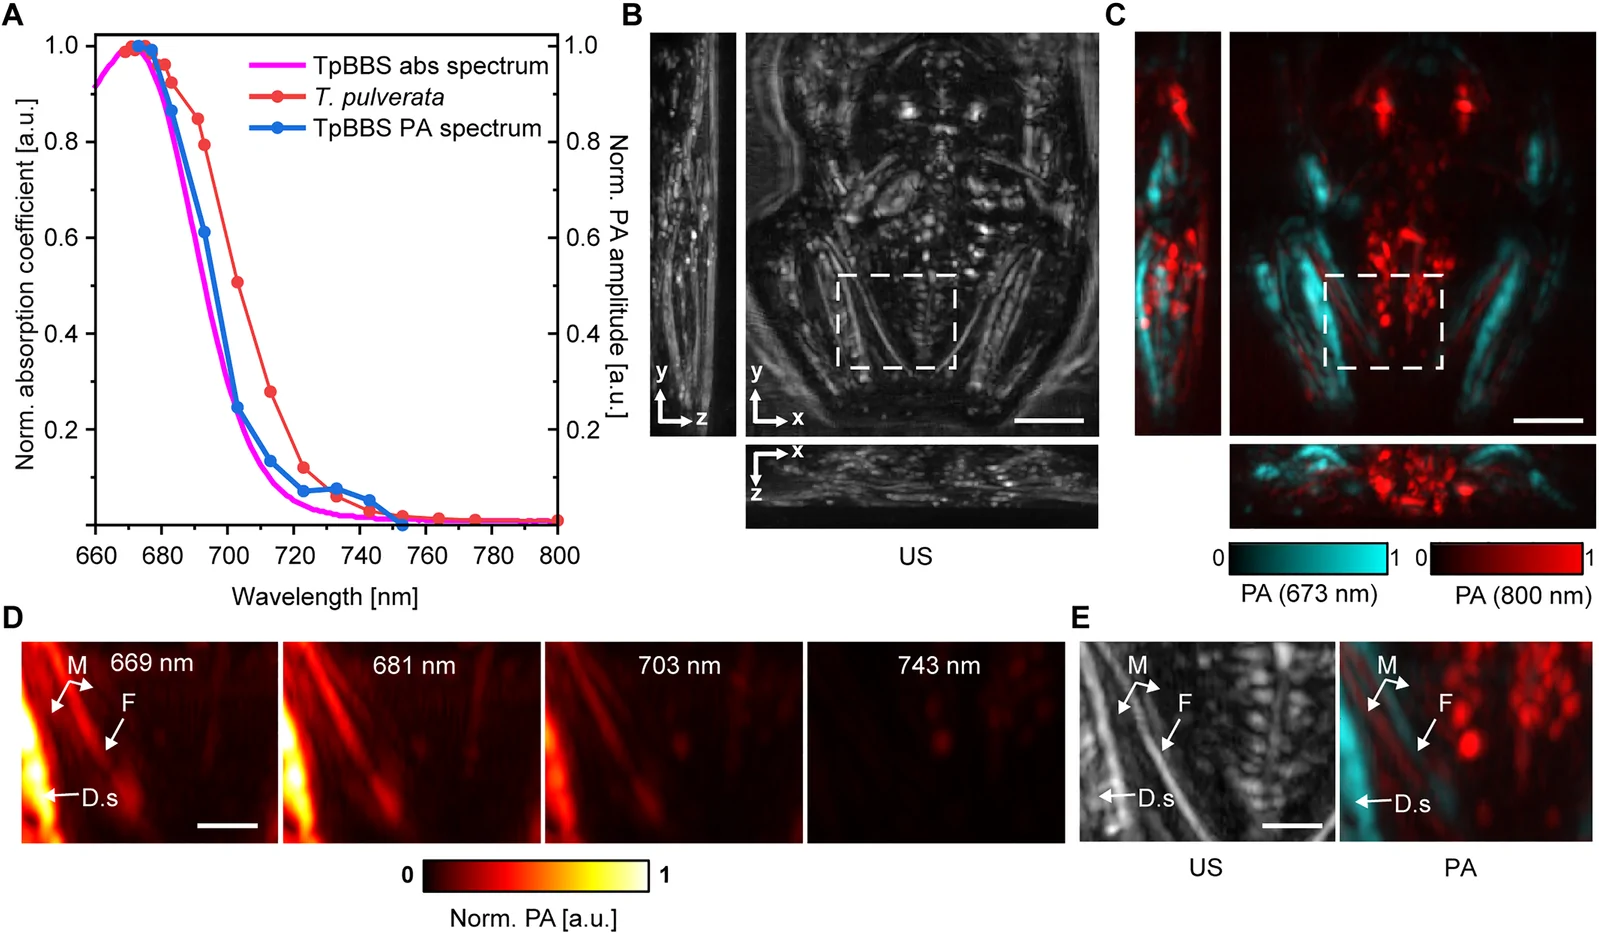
Hyperspectral PAT of *T. pulverata* reveals the presence of TpBBS in subcutaneous tissues. (A) Average PA amplitude of live frog (blue) and TpBBS-filled tube (pink) compared with the TpBBS’ optical absorption spectrum (red). (B) Referenced ultrasound (US) image of a sleeping frog, projected along three different dimensions, and (C) corresponding overlay of PA images showing TpBBS with 673-nm PA signal (light blue) and other pigments such as hemoglobin and melanin at 800 nm (red). (D and E) Zoomed-in region of the US and PA images at increasing wavelengths (D) and US and PA at 673 and 800 nm, respectively (E). TpBBS is distributed across multiple tissues, with high concentrations in the bones [femur (F)], lymph, dorsal skin (D.s.), and muscles (M). Because the internal organs of glassfrogs are covered by broadband reflectors that backscatter most of the incident light, the light fluence is attenuated in the dorsal skin immediately overlying the internal organs, which results in weak PA signals. Scale bars, 5 mm (B and C) and 2 mm (D and E).
